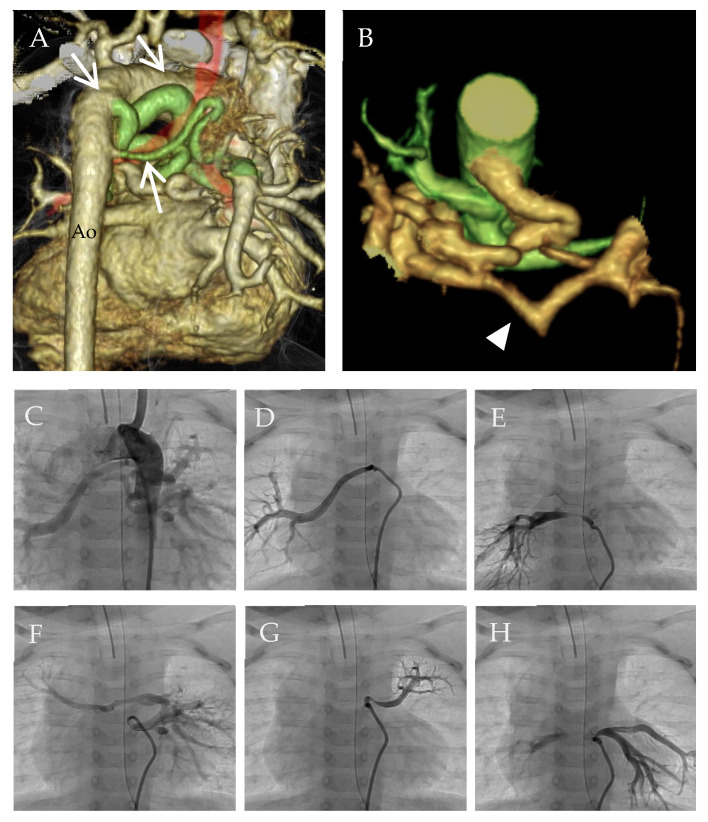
MDCT imaging and aortogram of MAPCA. (A) MAPCA (arrows) viewed from the back. (B) The central PA (arrowhead) and MAPCA. (C) Aortogram showing the total image of MAPCA. (D–H) Selective angiograms of MAPCA. Ao, descending aorta; MAPCA, major aorto-pulmonary collateral arteries; MDCT, multidetector computed tomography; PA, pulmonary artery.
